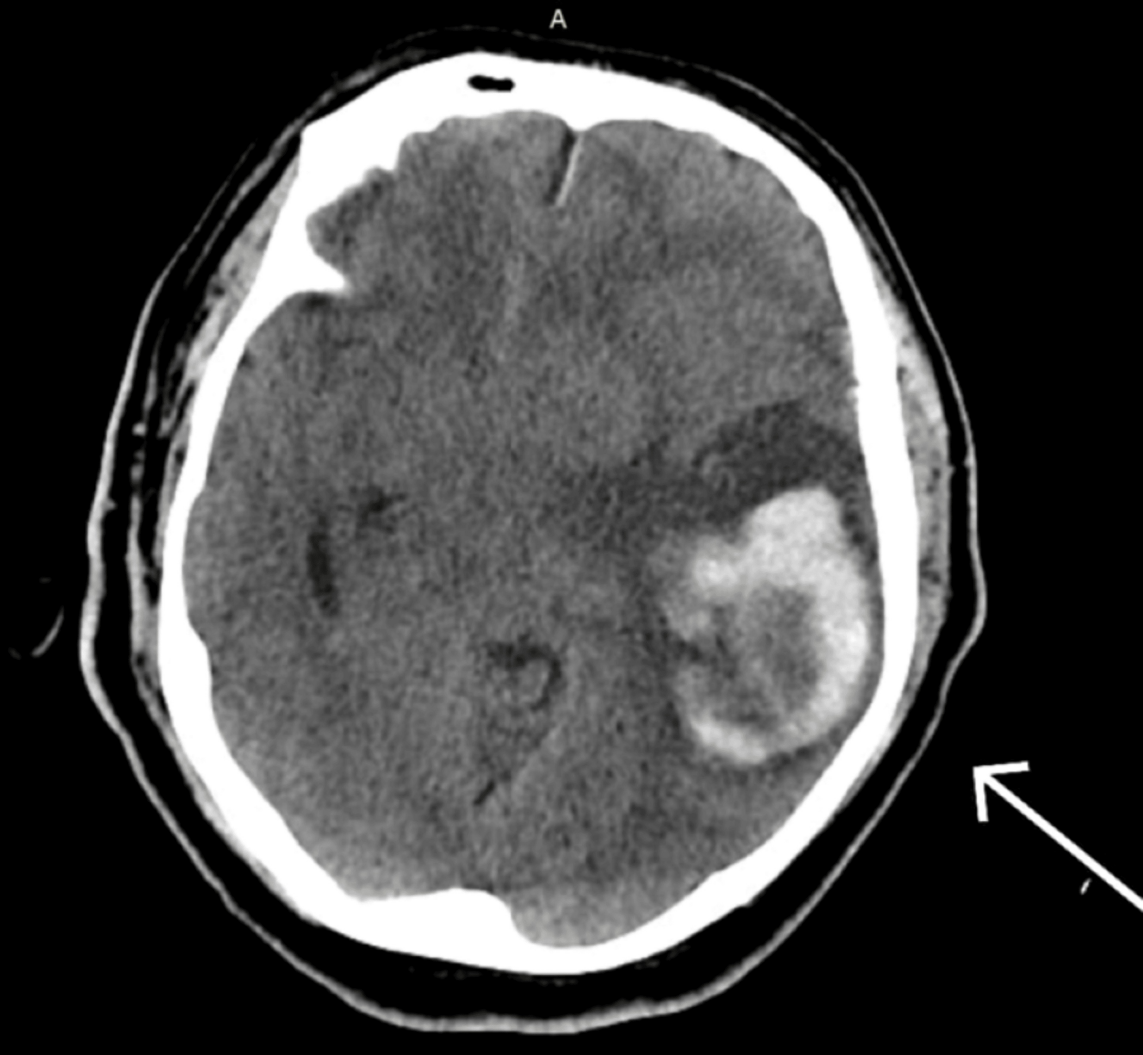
Initial presentation CT brain, with the arrow representing hemorrhagic area.
